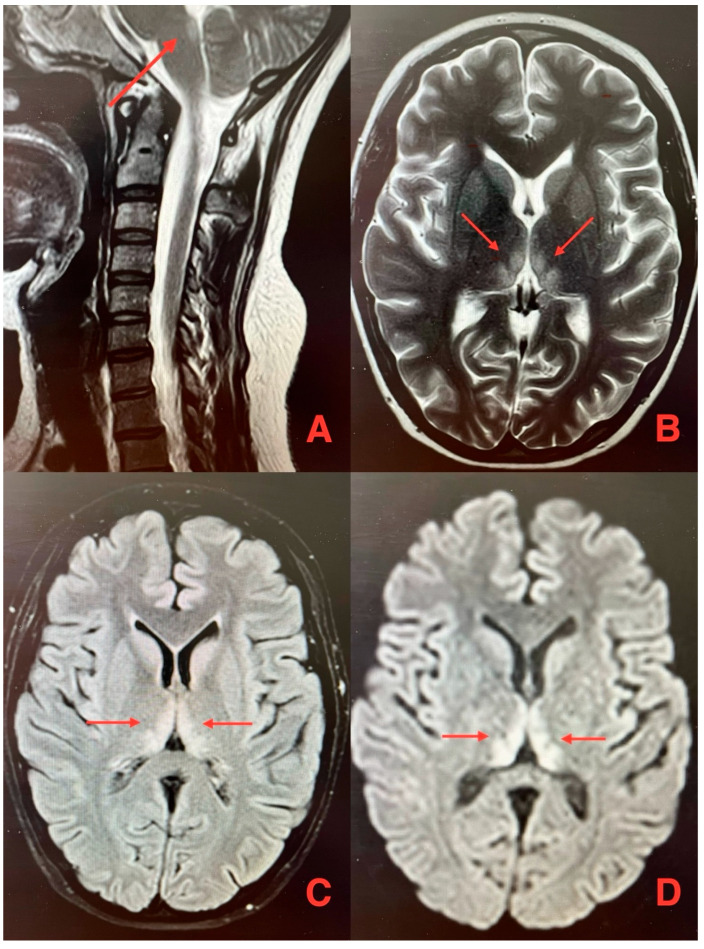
Magnetic resonance images of the brain show hyperintense lesions in the area of the third ventricle–arrows (T2-weighted sequence) (A) and in the medial area of both thalamuses (T2-weighted sequence (B), FLAIR sequence (C), and DWI sequence (D)) (author’s archive).
